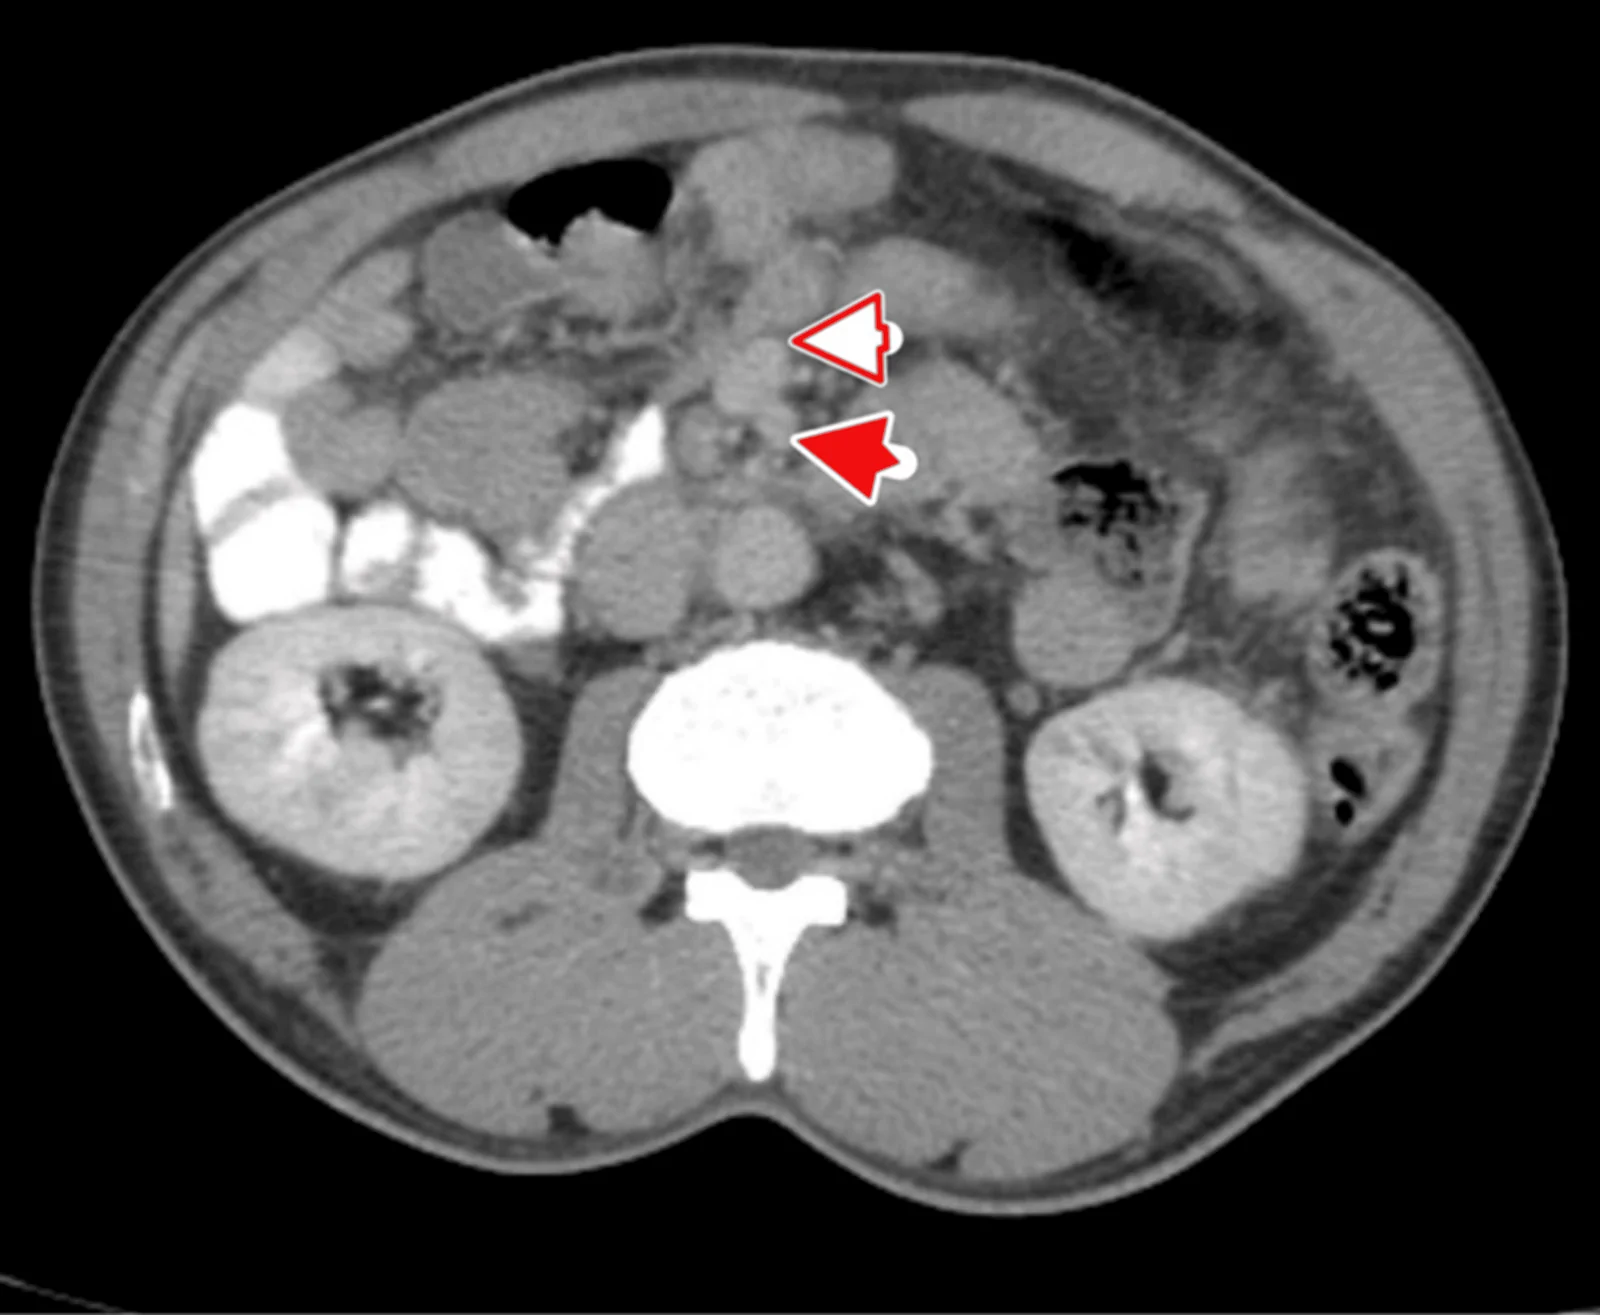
Intestinal nonrotation on axial CT Axial contrast-enhanced CT image demonstrating an inverse superior mesenteric artery (SMA)/superior mesenteric vein (SMV) relationship, with the SMV (arrowhead outline) located anterior to the SMA (arrowhead tapered), consistent with intestinal malrotation.
